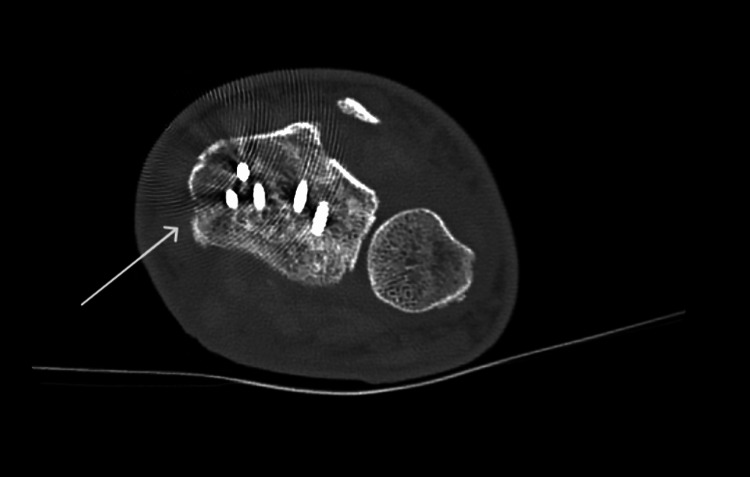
Postoperative CT following open reduction and internal fixation (ORIF) of the right distal radius with a volar locking plate. Arrows highlight plate position and fracture alignment.
